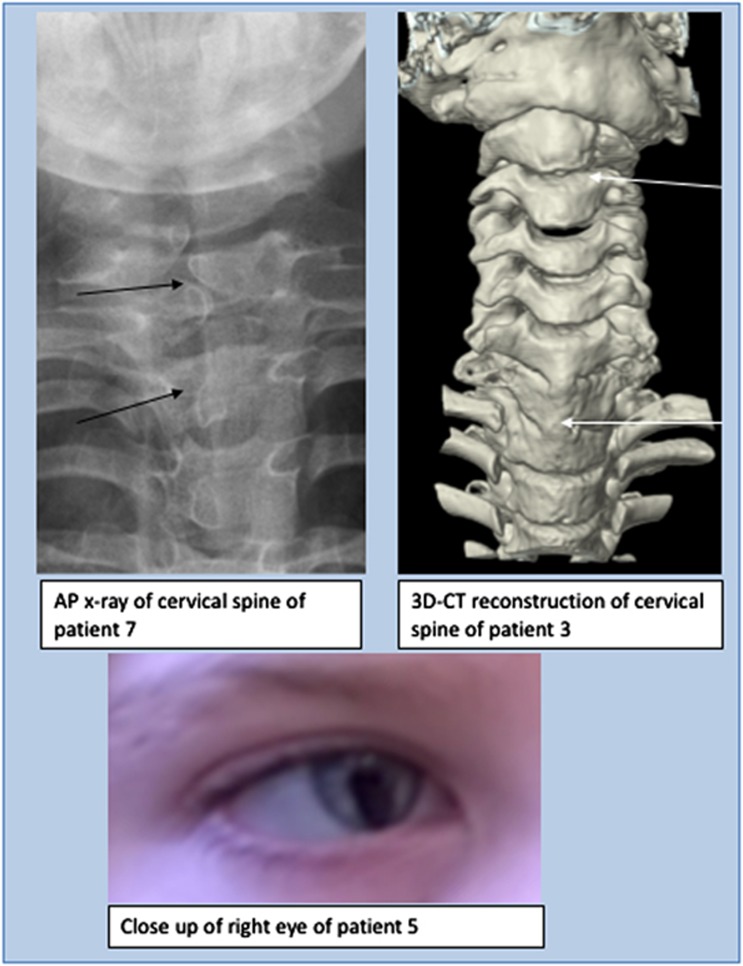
Spinal features (above): AP x-ray of cervical spine of patient 7 demonstrating hemivertebrae (left) and 3D-CT reconstruction of cervical spine of patient 3 demonstrating abnormalities of articulation of atlas with C1, vertebral bodies of C2/C3 and fusion of bodies of C6/C7/T1 and ocular features (below): right iris coloboma in patient 5.
